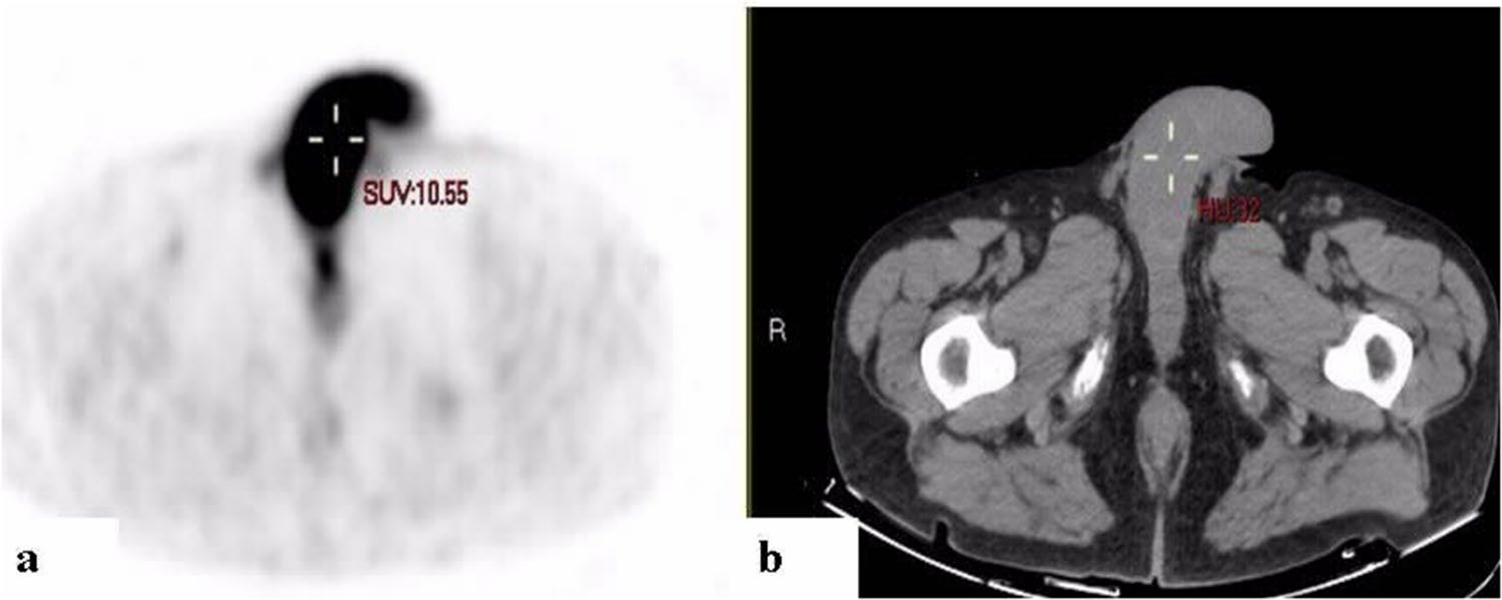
18F-FDG PET (a) and CT (b) axial slices demonstrating diffusely increased uptake along the penis. A 73-year-old man with relapse of diffuse large B-cell lymphoma (patient #16, Table 1).
